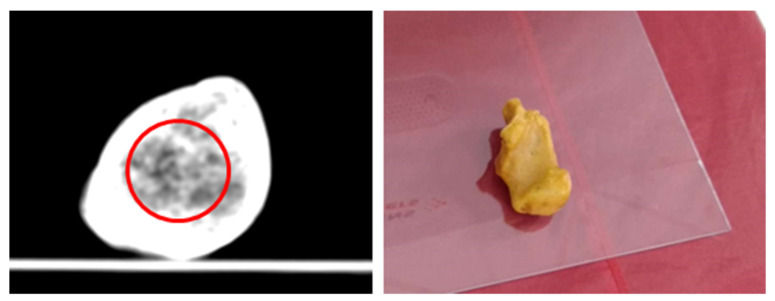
The CT scanned calcaneus bone (left) and the calcaneus bone placed on the CT patient bed (right).
